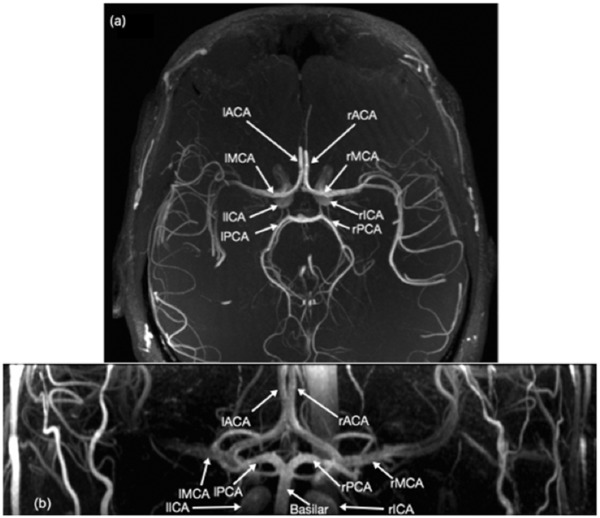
Time‐of‐flight magnetic resonance images of the circle of Willis at 7 T from a cranial (a) and a dorsal (b) view. Abbreviations: l/rACA, left/right anterior cerebral artery; l/rICA, left/right internal carotid artery; l/rMCA, left/right middle cerebral artery; and l/rPCA, left/right posterior cerebral artery
